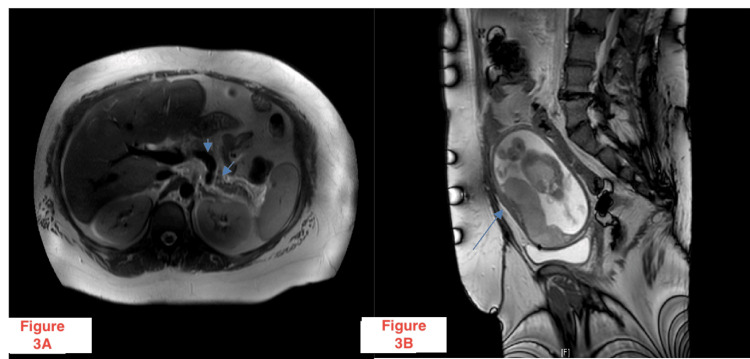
MRI abdomen/pelvis axial (3A) and sagittal (3B) images demonstrating edema of the pancreas with peripancreatic stranding and fluid compatible with pancreatitis.
